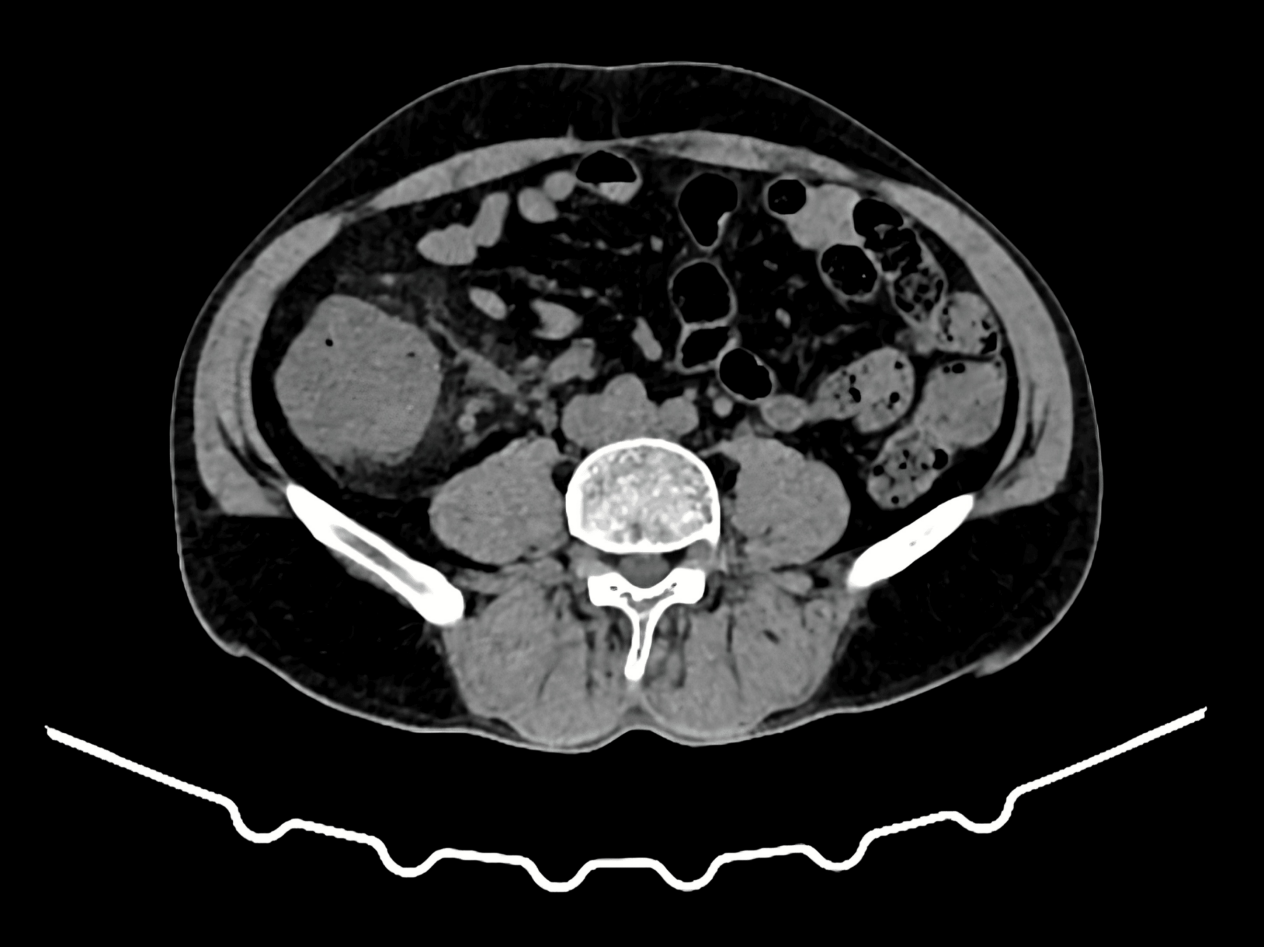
CECT scan revealed a 7 cm long segment intussusception of the terminal ileum into the caecum and ascending colon, with the mesentery and its vasculature CECT: contrast-enhanced computed tomography
